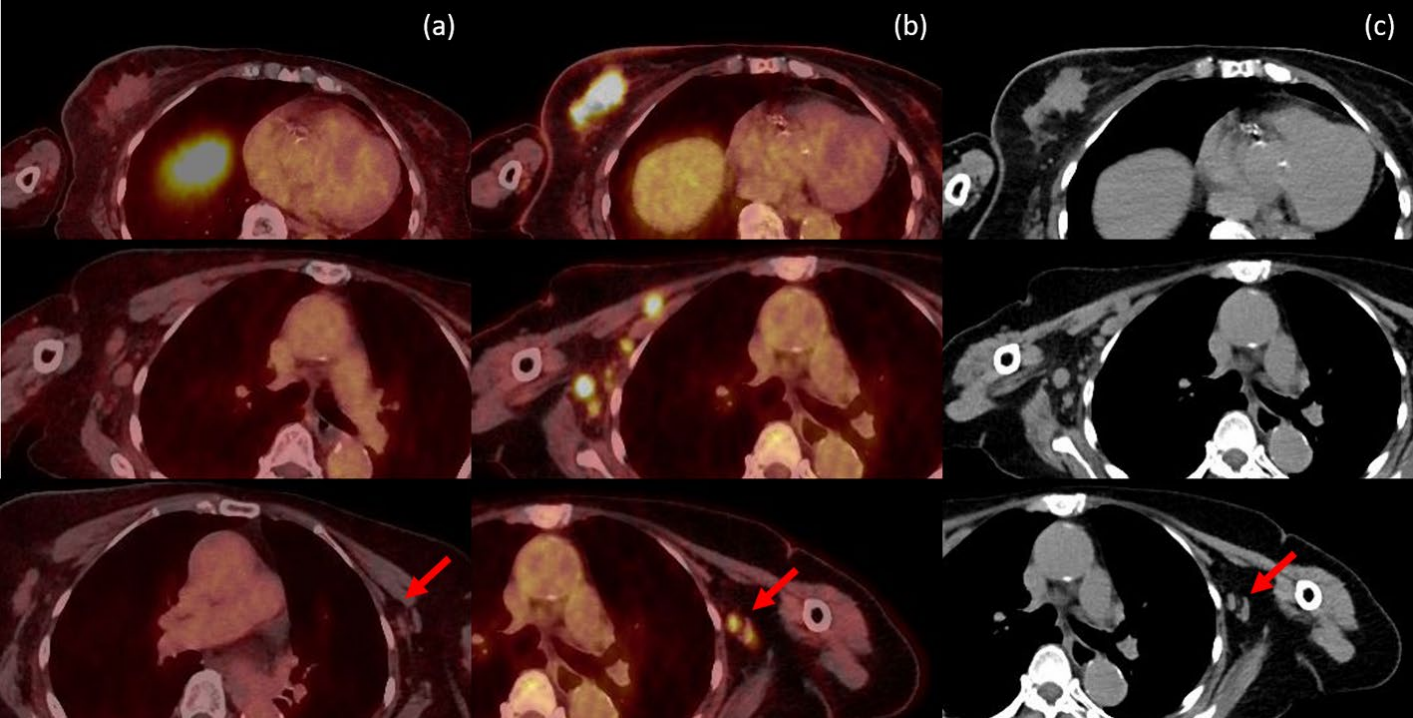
Fusion PET axial images of ^18^F-FES a and ^18^F-FDG b for ^18^F-FDG-avid right breast mass and multiple enlarged metastatic nodes at right axilla on CT imaging c without ^18^F-FES avidity, in line with few small ^18^F-FDG-avid nodes at left axilla (arrow) but no ^18^F-FES avidity compatible with reactive nodes from recent vaccination and decreased size in follow-up imaging
